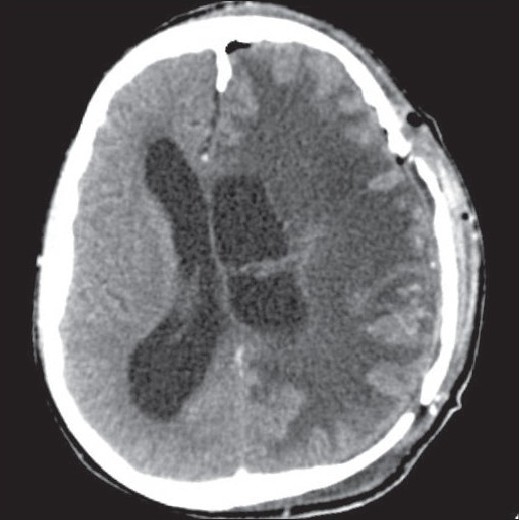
Postoperative contrast-enhanced computed tomography scan image showing no residual tumor
